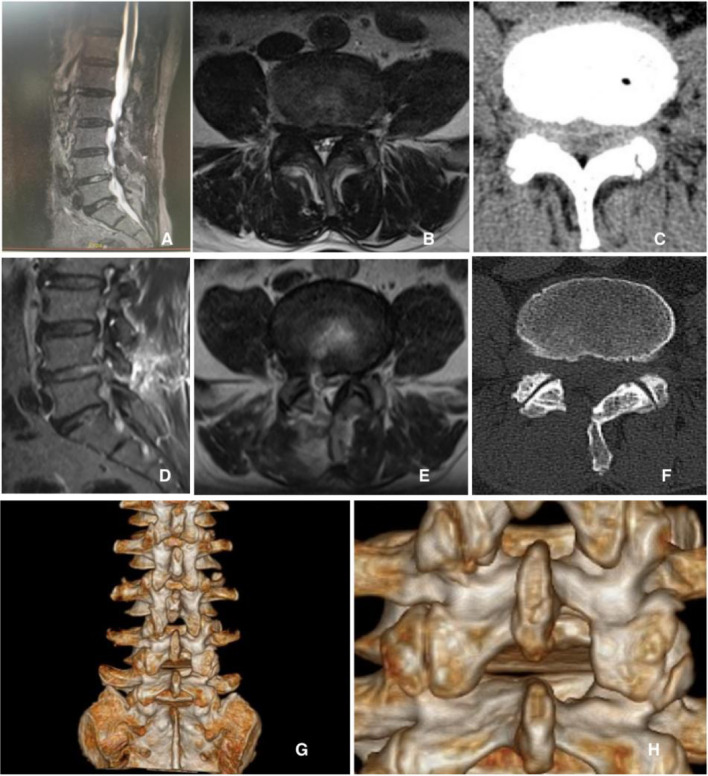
Pre-and postoperative radiologic images of the sixth case. A 58-year-old man presented with lateral recess and cranial level foraminal stenosis on the L4–L5 level. He underwent UBE-CIA on the right side of the L4–L5 level. Preoperative images showed foraminal stenosis on the right side of the L4–L5 level (A–C); ideal foraminal decompression with an obliquely undercut facet joint was shown in postoperative images (D–F); and three-dimensional computed tomography scan identified the remained facet joints and the range and adequacy of foraminotomy (G, H).
